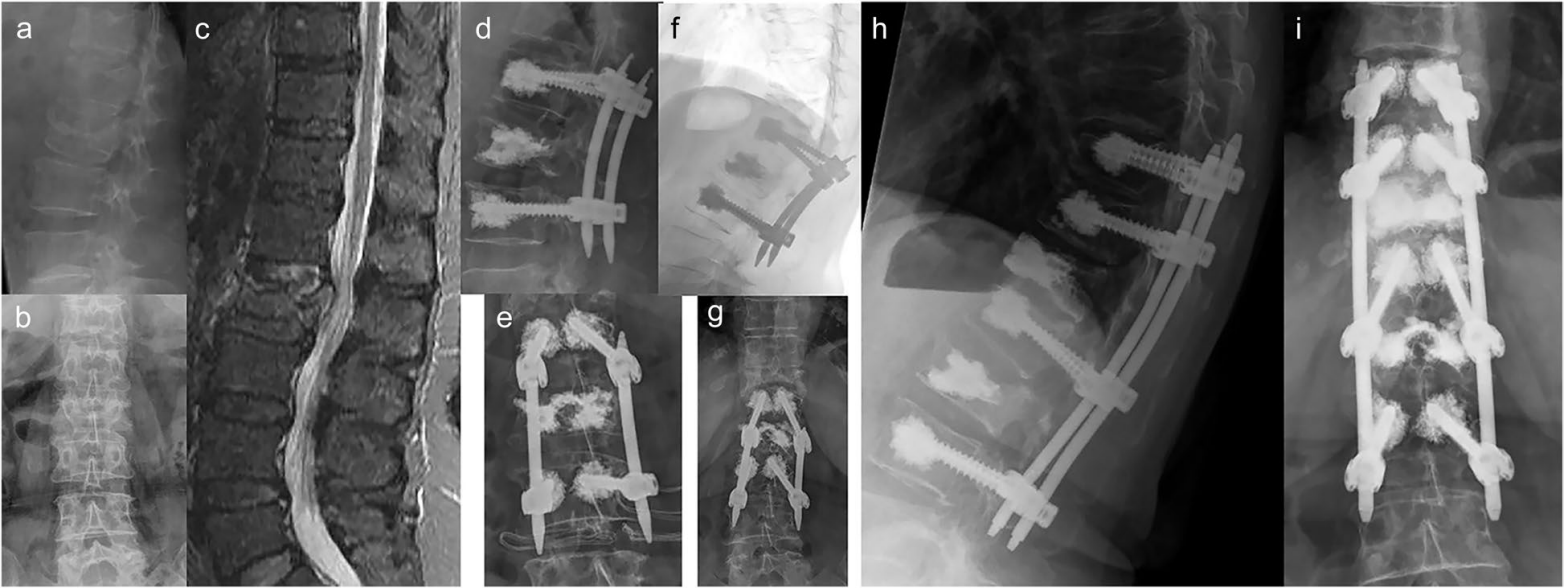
A case with a subsequent vertebral fracture is presented. A 72 year old patient suffered from an acute L2 fracture type OF 4 while helping to lift her husband out of the bed 8 days ago a-c. This trauma mechanism was defined as not adequate. Based on the intense pain despite adequate analgetig medication hybridstabilization consisting of percutaneous short-segmental posterior stabilization and cement augmentation of the fractured vertebral body was performed d,e. Acute pain occurred four months after having an eventful initial course. There was no trauma history. The radiographs depicted an subsequent fracture of the thoracic vertebral body 12 with kyphotic malalignment f,g. This was treated with long-segmental stabilization and cement-augmentation of the fractured vertebral body. The latest radiographs 6 years after the initial fracture are shown h,i
